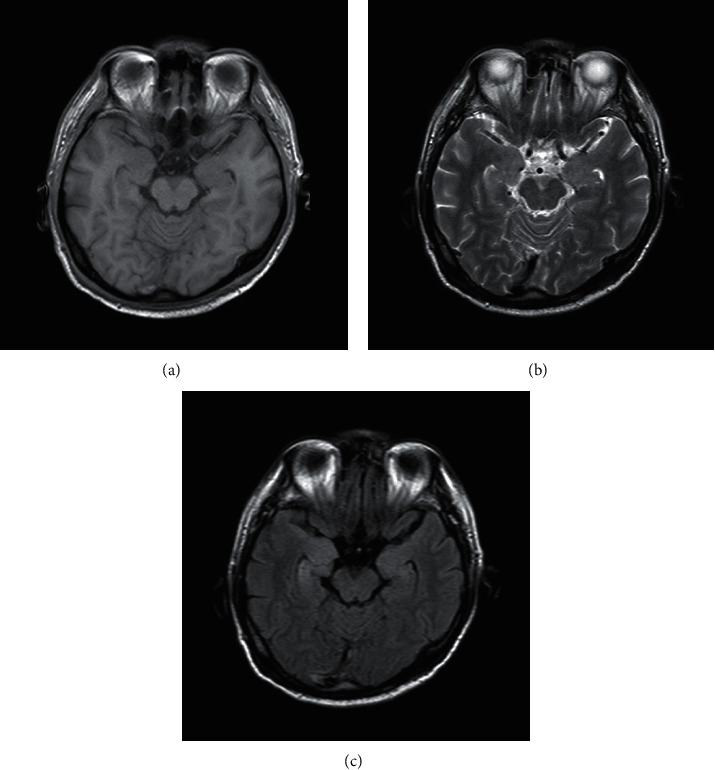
The cranial brain MRI of one case of anti-LG1 receptor encephalitis: (a) bilateral long tabular hippocampal T1, (b) slightly longer signal T2, and (c) FLAIR sequence with a slightly higher signal and repeat brain MRI showed bilateral hippocampal swelling.
